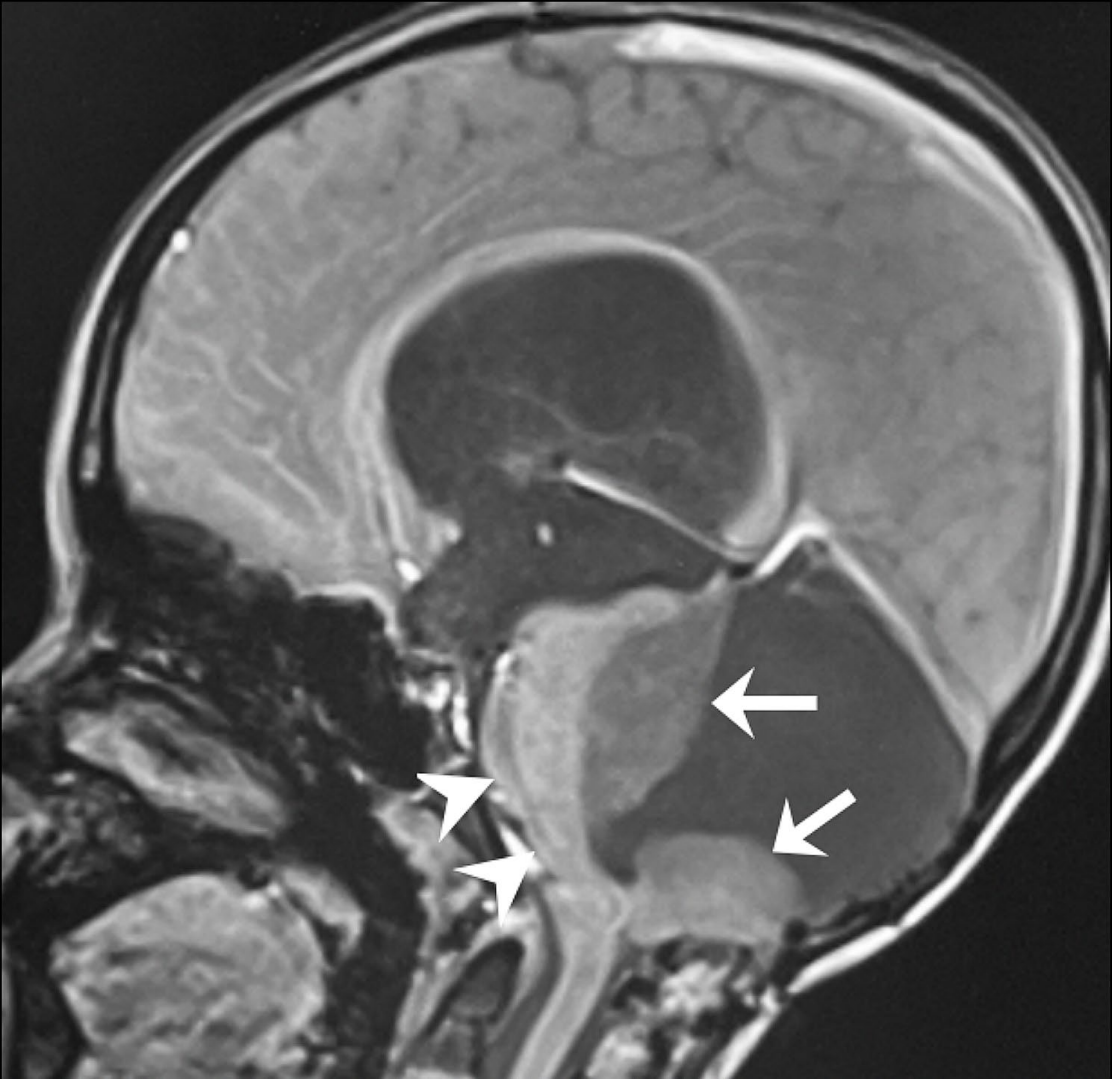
Magnetic resonance images (T1-weighted sagittal section) of brain before surgery. Ten hours before surgery. Arrows and arrowheads indicate cerebellar tumor and brainstem, respectively
